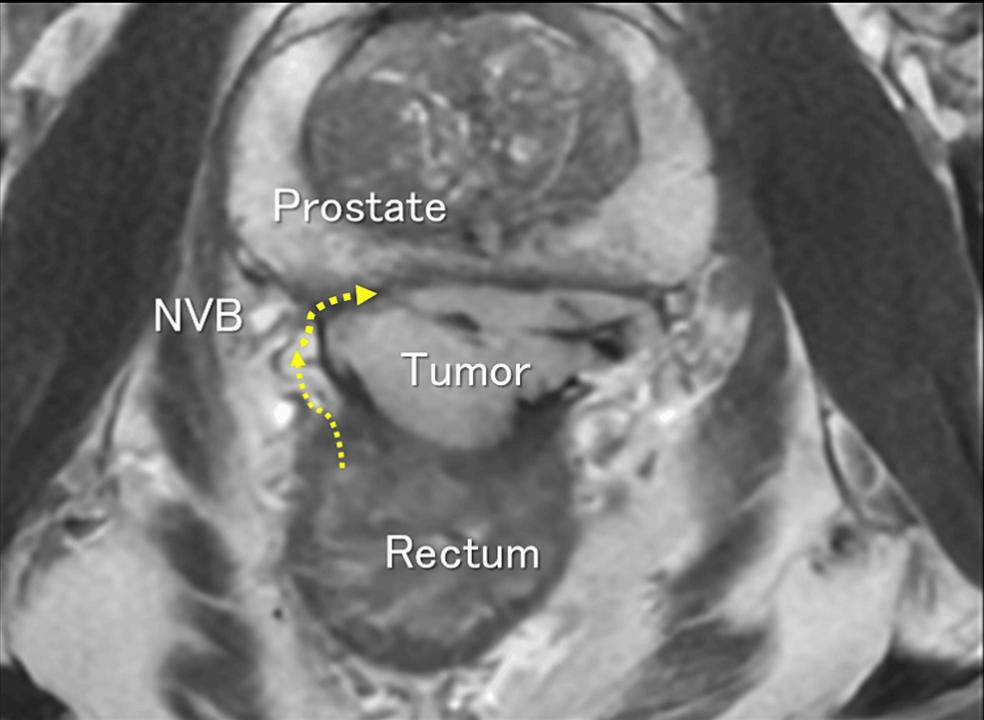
Strategy for surgery, identifying the prostate, the key extraluminal landmark On axial MRI, we planned to start with lateral dissection and identify the surface of prostate, the key extraluminal landmark (yellow arrow). NVB, neurovascular bundles
